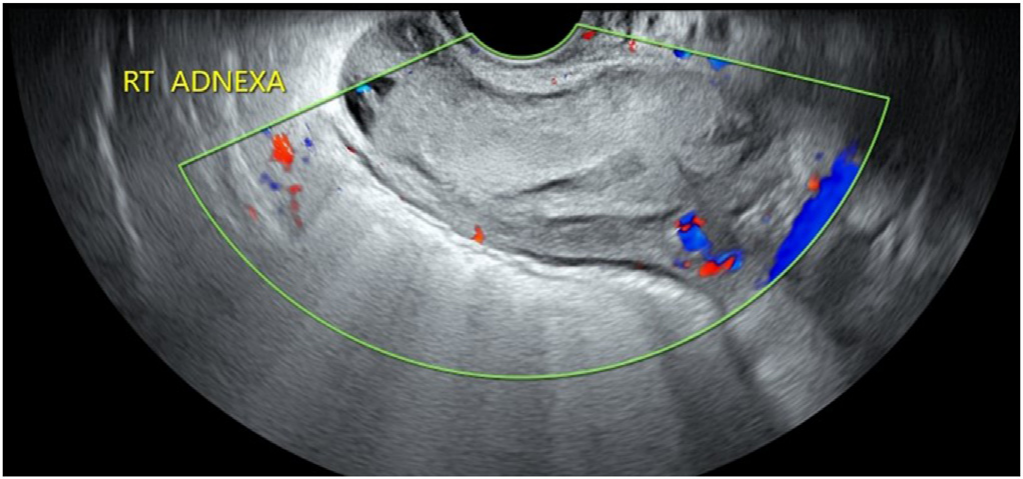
Transvaginal ultrasound using color Doppler demonstrates a nonhomogeneous mass in the right adnexa. Reprinted from content created by Dr. Salma Babikir Ahmed Erabi from Radiopaedia.org, rID:83742, under CC BY-NC-SA 3.0 license (https://creativecommons.org/licenses/by-nc-sa/3.0).
